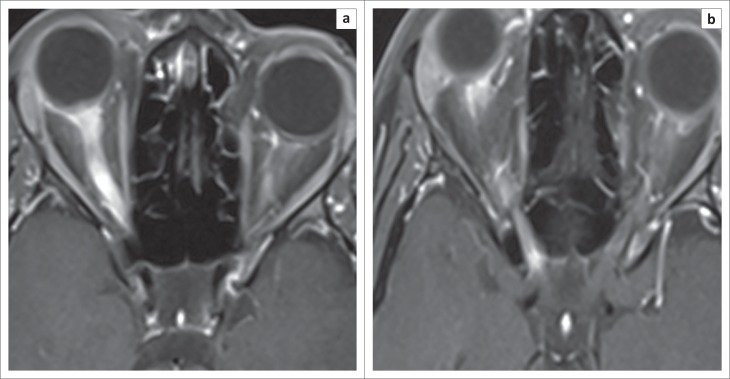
a) and (b) are orbital MRI views post-contrast. They show diffuse enhancement of the right optic nerve extending to the chiasm (arrows). Minimal enhancement of the left optic nerve is also present (right arrow in (b)).
